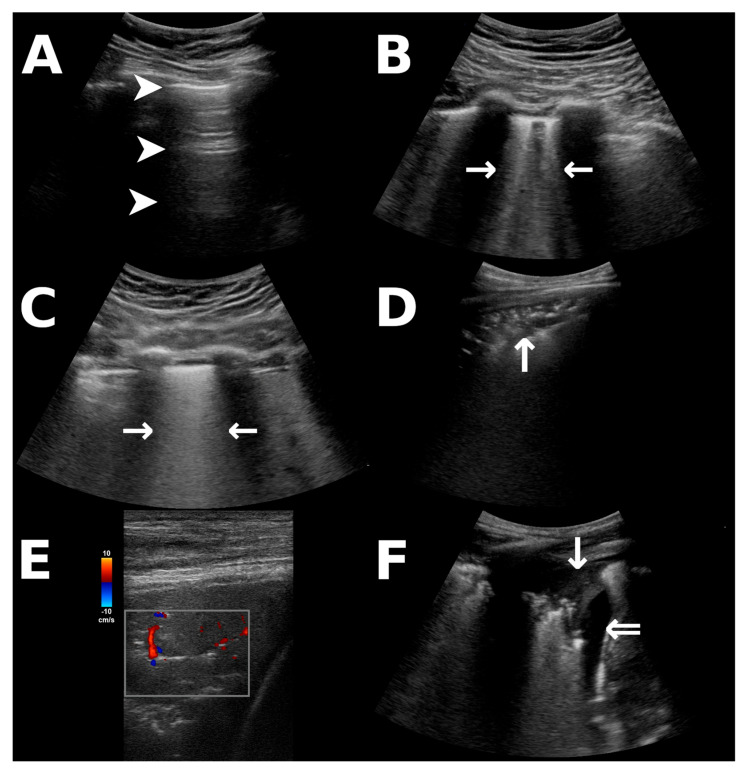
Ultrasonographic findings that were incorporated into the scoring system. All images were obtained from patients in our study population. (A) A-lines as reverberations of the pleural line (arrowheads) indicate fully aerated lungs or pneumothorax, distinguishable by dynamic pleural gliding [3,4]. (B,C) B-lines (horizontal arrows) arising from the pleural line indicate interstitial pathology and appear distinct (B) or merge to form coalescent B-lines (C), the latter corresponding to alveolar edema [4]. (D) Peripheral consolidations (vertical arrow) appear tissue-like and may contain hyperechoic air bronchograms. (E) Color Doppler ultrasonography of a pulmonary consolidation (red: flow towards the ultrasound probe, blue: flow away from the probe; scale for reference) in COVID-19 may demonstrate diminished pulmonary perfusion [1]. (F) Atelectatic lung (vertical arrow) within a pleural effusion (double arrow).
